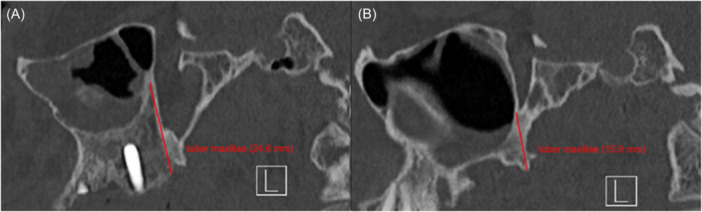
Sagittal skull CT scan of a patient suffering from SSS, (A) Tuber maxillae height of the SSS‐affected side (right maxillary sinus), (B) tuber maxillae height of the healthy side (left maxillary sinus). CT, computed tomography; SSS, silent sinus syndrome.
